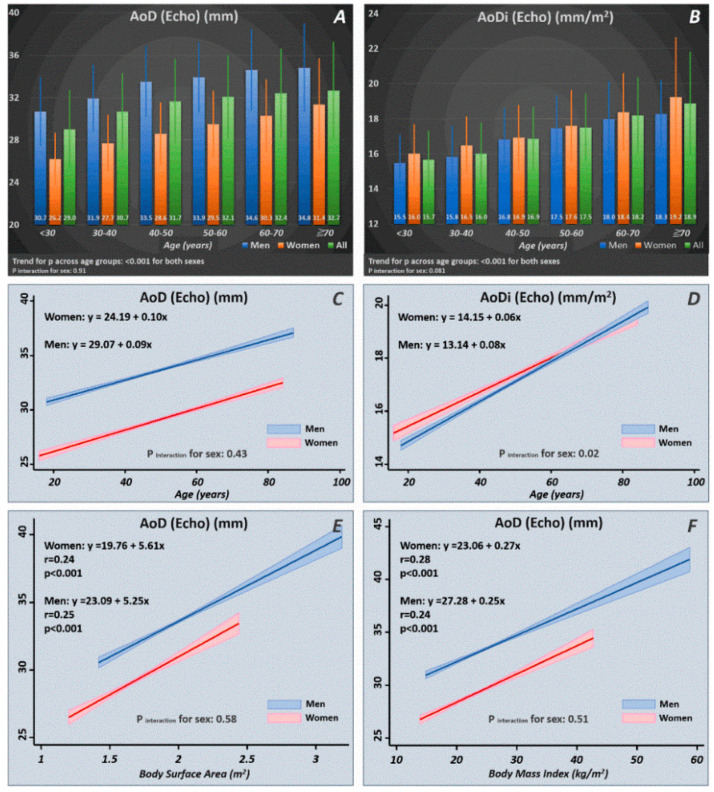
Normal reference ranges of AoD/AoDi by age and sex strata (A,B) drawn from our healthy participants (*n* = 3646). Linear relationships of AoD/AoDi with increasing age (C,D) and larger body size in terms of body surface area (BSA) and body mass index (BMI) (E,F) in men and women. AoD: aortic root diameter; AoDi: indexed aortic root diameter; Echo: echocardiography.
